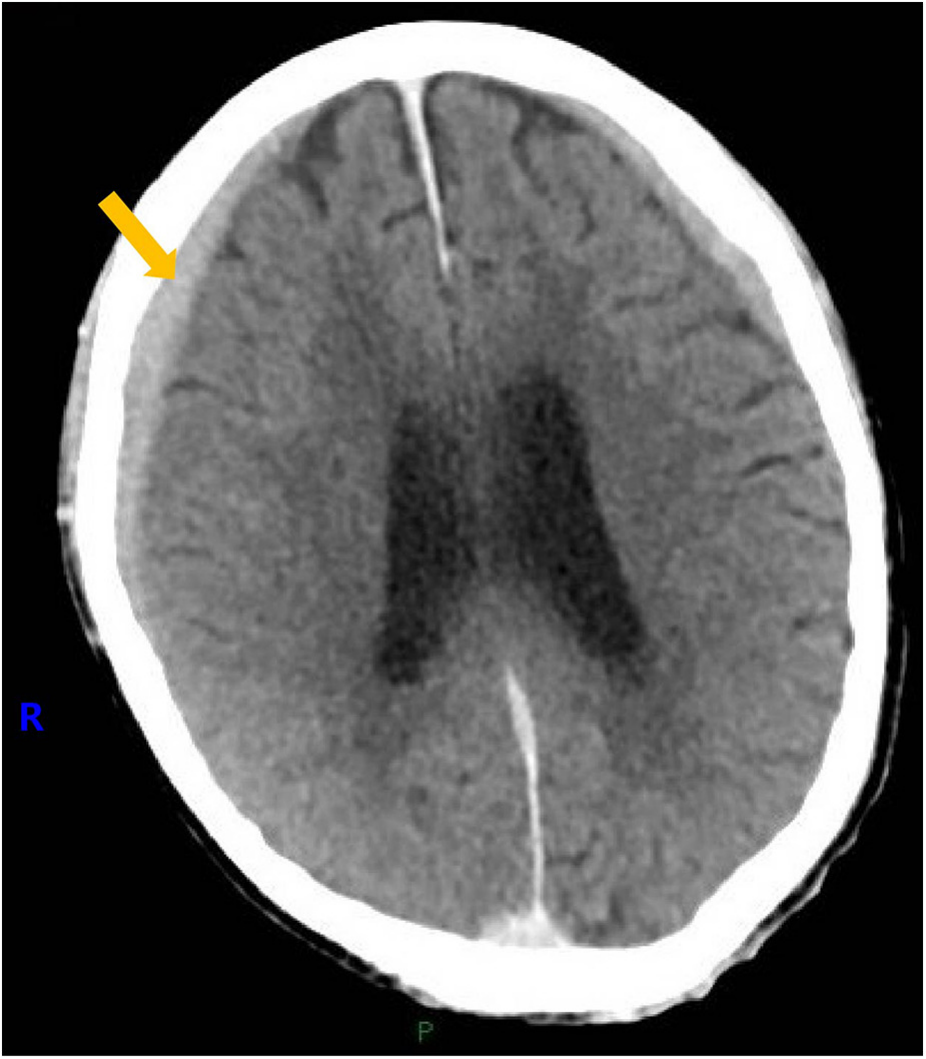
Cranial computed tomography image of a thin subdural hematoma in the right frontal and temporal region.
